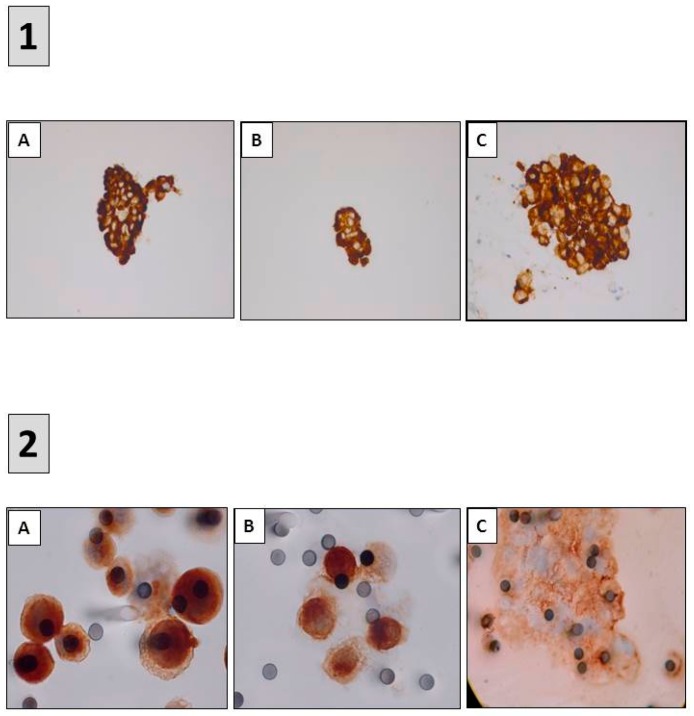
Detection of predictive biomarkers using immunocytochemistry (ICC). (1) ALK ICC (D5F3) (A) ROS1 ICC (D4D6) (B) and PD-L1 ICC (22C3) (C) performed on samples obtained after endobronchial ultrasound trans-bronchial needle aspirations (A–C) immunoperoxidase, magnification ×400. (2) ALK ICC (D5F3) (A) MET ICC (SP44) (B) and PD-L1 ICC (22C3) (C) performed on circulating tumor cells isolated on filters after blood filtration (A–C) immunoperoxidase, magnification ×1000.
